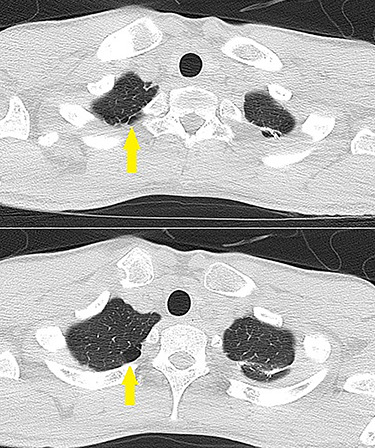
Lung CT showing bullae on the pulmonary apex of the left lung (arrows).
